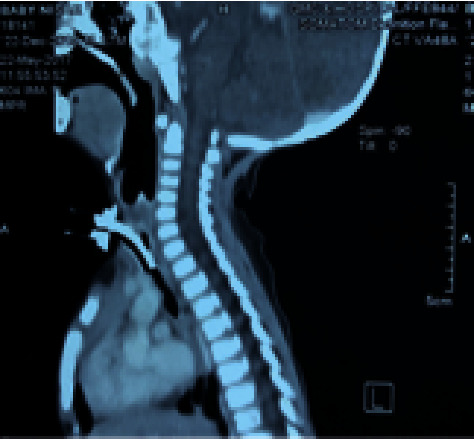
Preoperative sagittal CT image with tracheostomy tube in situ.
